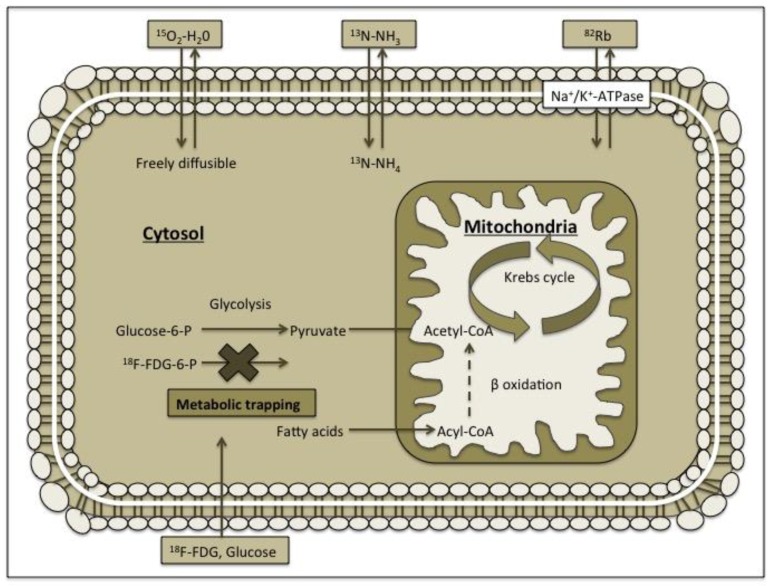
Mechanism of action of different positron-emission tomography (PET) tracers. Fludeoxyglucose (FDG) is a biological analogue of glucose but unlike glucose it is intracellularly trapped and does not undergo further metabolism. ^15^O-water is metabolically inert and freely diffusible perfusion tracer. ^82^Rb is the perfusion tracer that is taken up by myocardium as a potassium analogue through the active transport by the Na+/K+ ATPase-pump. ^13^N-NH_3_ is taken up by the myocardium by passive free diffusion across cell membranes as ammonia (NH_3_) where it equilibrates with its charged form ammonium (NH_4_).
